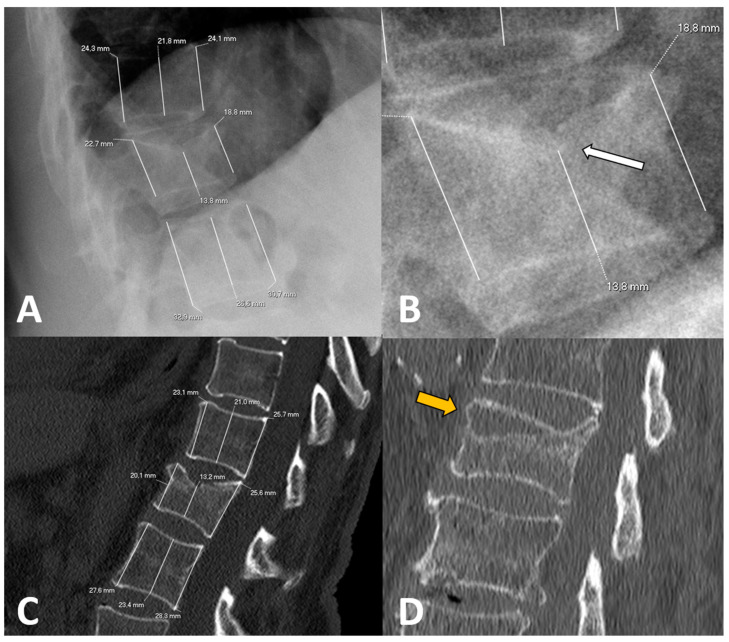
Examples of radiological variables assessed in our sample, from a 58-year-old woman (A–C) and an 88-year-old man (D). Patient 1. X-rays ((A), magnified in (B)) and CT at diagnosis (C). The height of each wall (anterior, middle and posterior) was measured through lines parallel to the anterior vertebral walls. Relative measurements were obtained for each fractured vertebral body by dividing them between the mean of the respective measurements in the cephalad and caudal vertebral bodies. Note that some cases involved subtle findings such as the asymmetric, focal endplate depression depicted with a white arrow in (B). Patient 2. CT at diagnosis (D) showing an obvious subchondral defect (orange arrow) consistent with a large intravertebral cleft.
